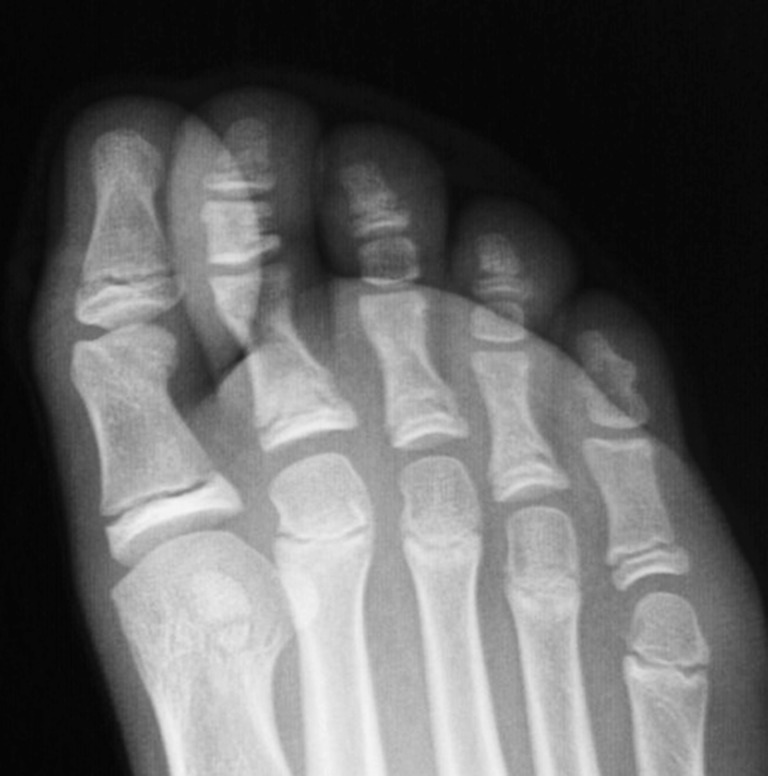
Foot radiograph of a 12-year-old female with complete fusion of the distal phalanx of a biphalangeal 5th toe, and incomplete fusion of the 1st–4th toe
